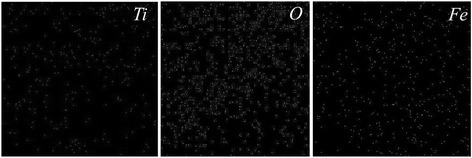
Kα X-ray mapping of Fe, O, and Ti over the surface of N/Fe_x_Ti_y_O_z_ film treated at 450 °C
